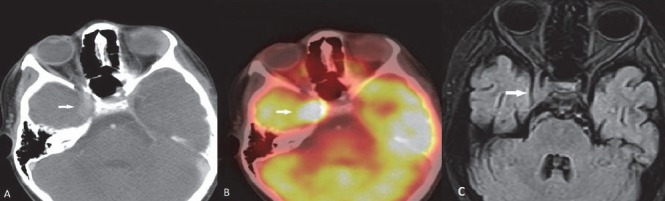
Burkitt’s lymphoma involving CNS: Right cavernous sinus shows enhancing deposit (A) with FDG avidity (B). T2W axial scan of MRI brain through same plane shows soft tissue deposit isointense to gray matter in the region of right cavernous sinus
